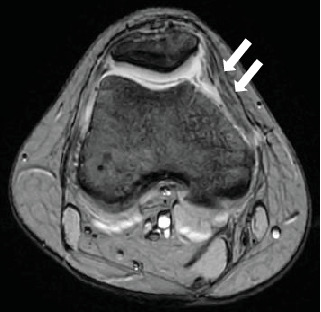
MRI image showing the reconstructed MPFL, as indicated with the arrow.
